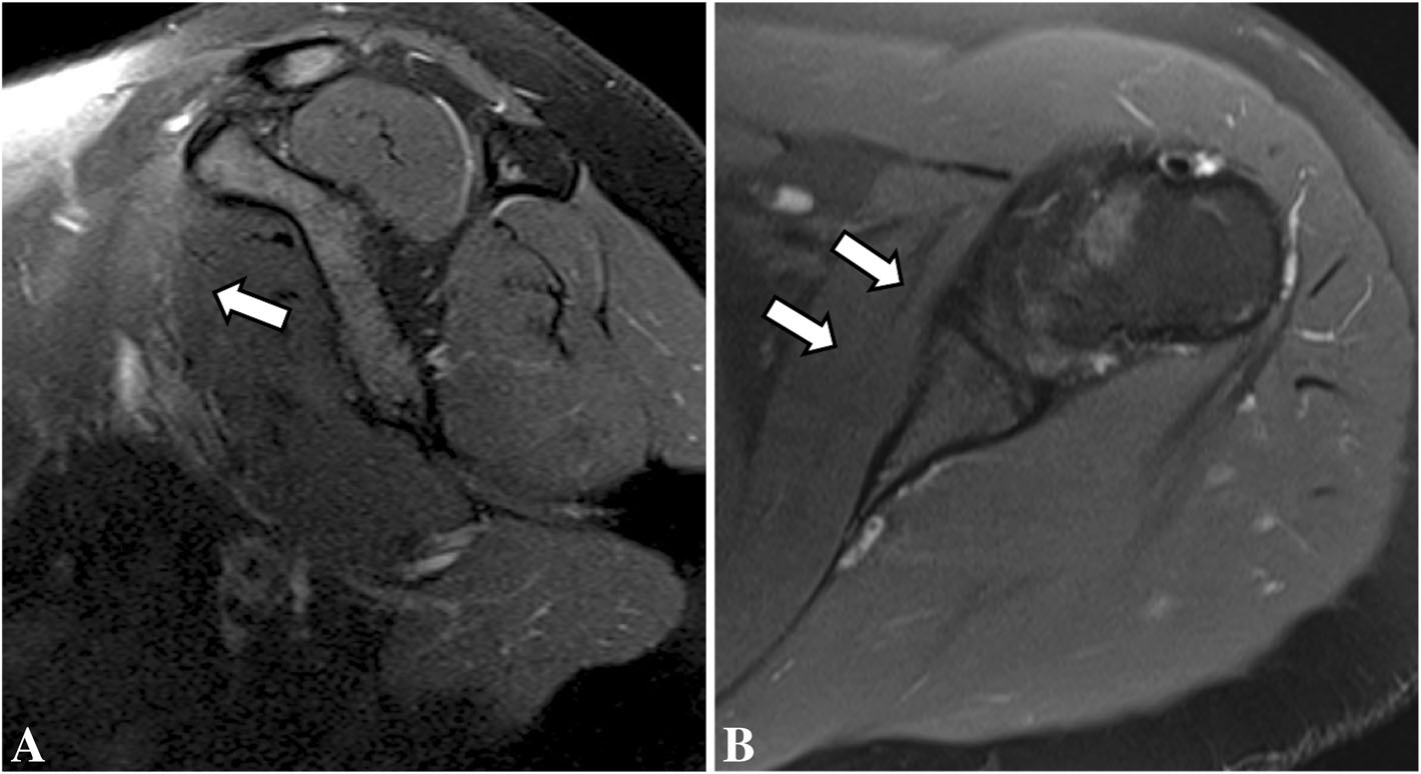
Sagittal (a) and axial (b) T2-weighted fat-suppressed follow-up MR images showing resolution of the subscapularis muscle edema (arrows)
